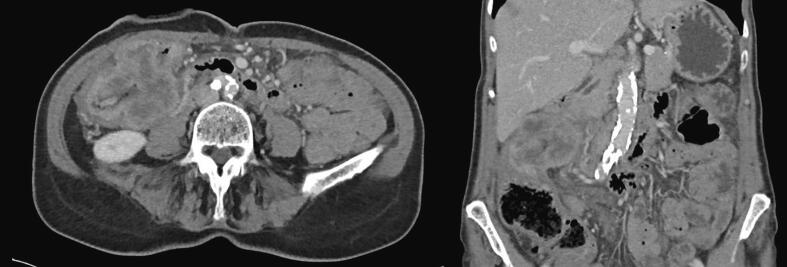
The CT scan before neoadjuvant therapy that shows an ascending colon tumor with suspected ingrowth into the duodenum and abdominal wall.
